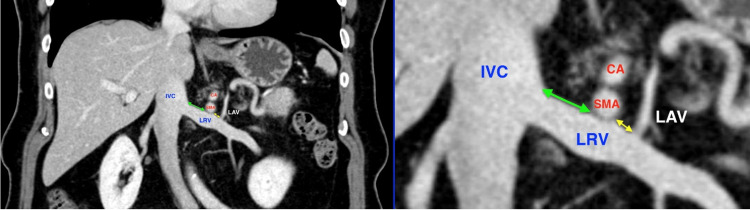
Coronal CT scan image showing the relationship between the LRV and the root of the SMA. The distance from the IVC to the root of the SMA is marked by the green arrow, and the distance from the LAV to the root of the SMA is marked by the yellow arrow. LRV: Left renal vein; SMA: superior mesenteric artery; CA: celiac axis; IVC: inferior vena cava; LAV: left adrenal vein; CT: computed tomography
